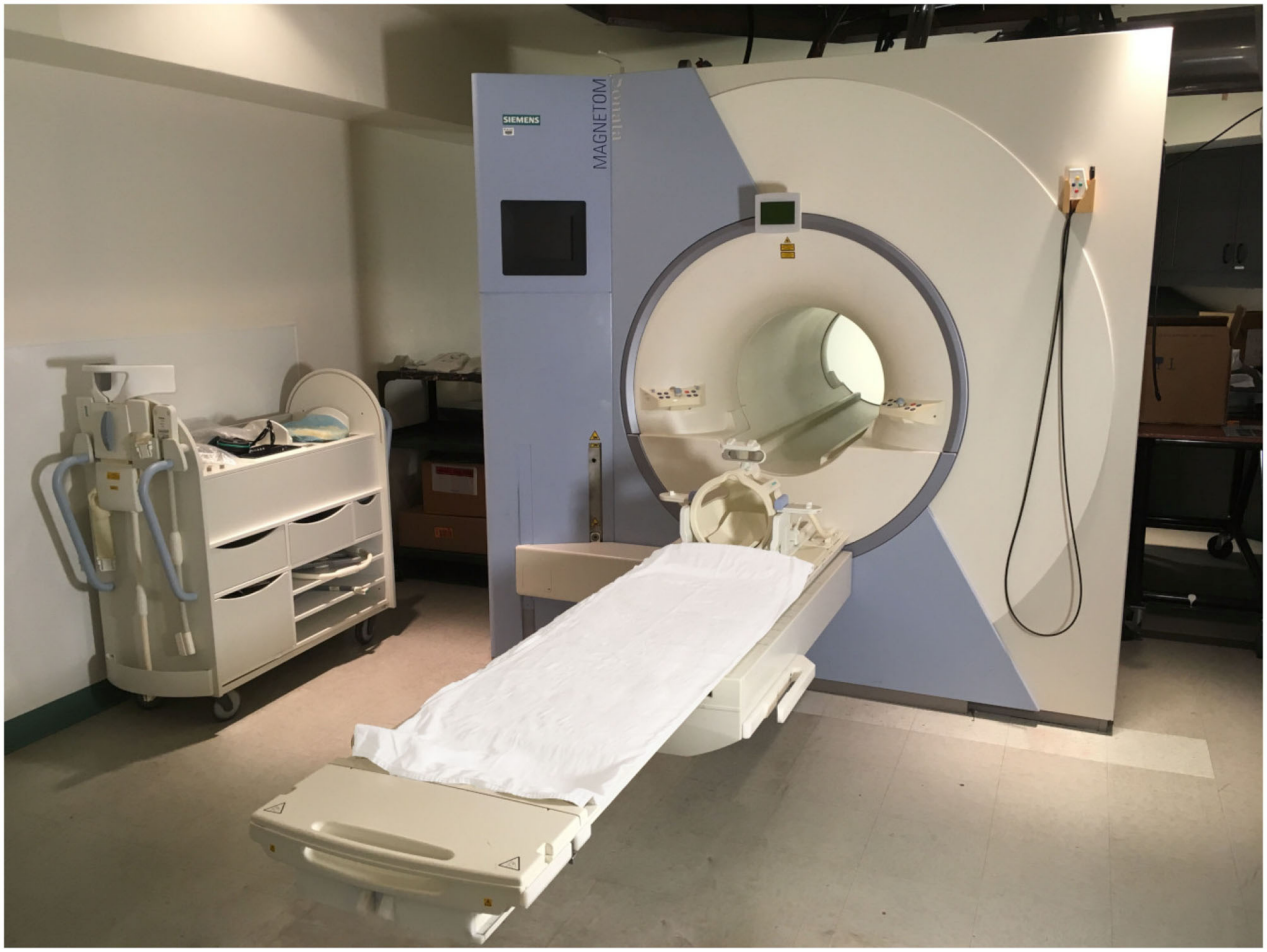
Decommissioned MRI scanner. The scanner appeared to function but the magnet was off.
